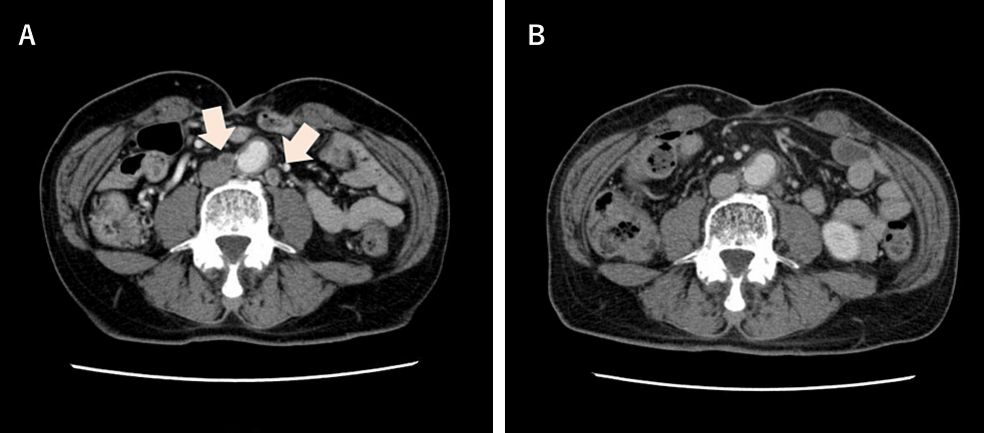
Contrast-enhanced computed tomography of the abdominal region Contrast-enhanced computed tomography of the abdominal region before 1st chemoradiotherapy (A) and after the chemoradiotherapy (B). The 0range arrow indicated swollen lymph nodes.
